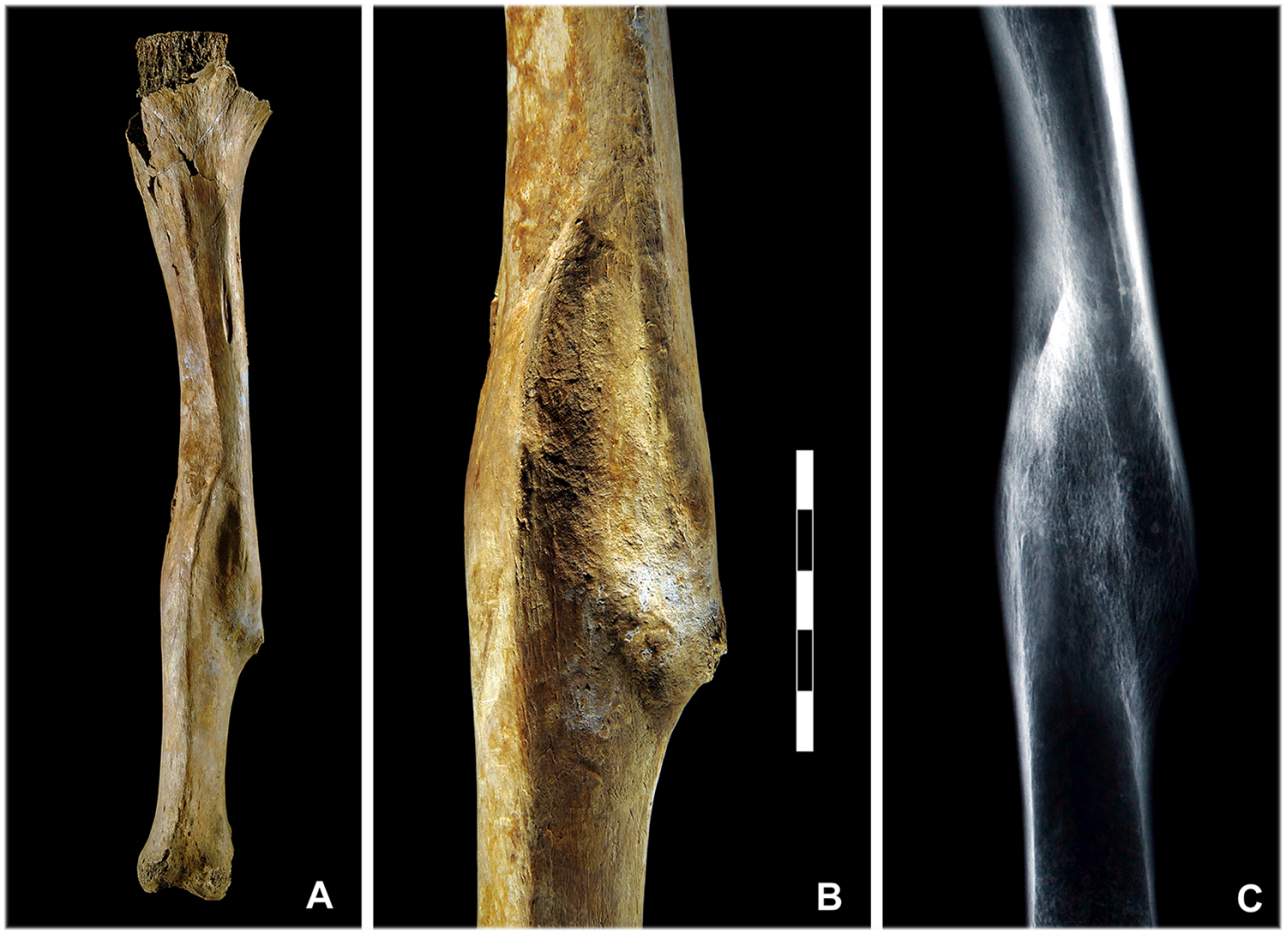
Healed long bone fracture. (A, B) The left tibia of individual 17 shows a healed fracture. (C) The fracture ends did not reunite correctly due to the clear malunion. However, the healed bone exhibits no evidence to suggest infection (photos: K. Bentele; radiograph: LDA Halle/Saale).
